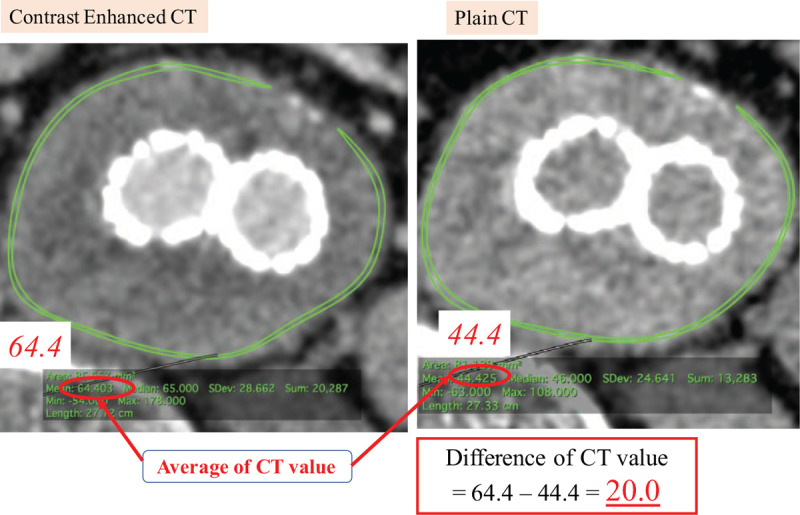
Definition of aortic wall enhancement. CT = computed tomography.
